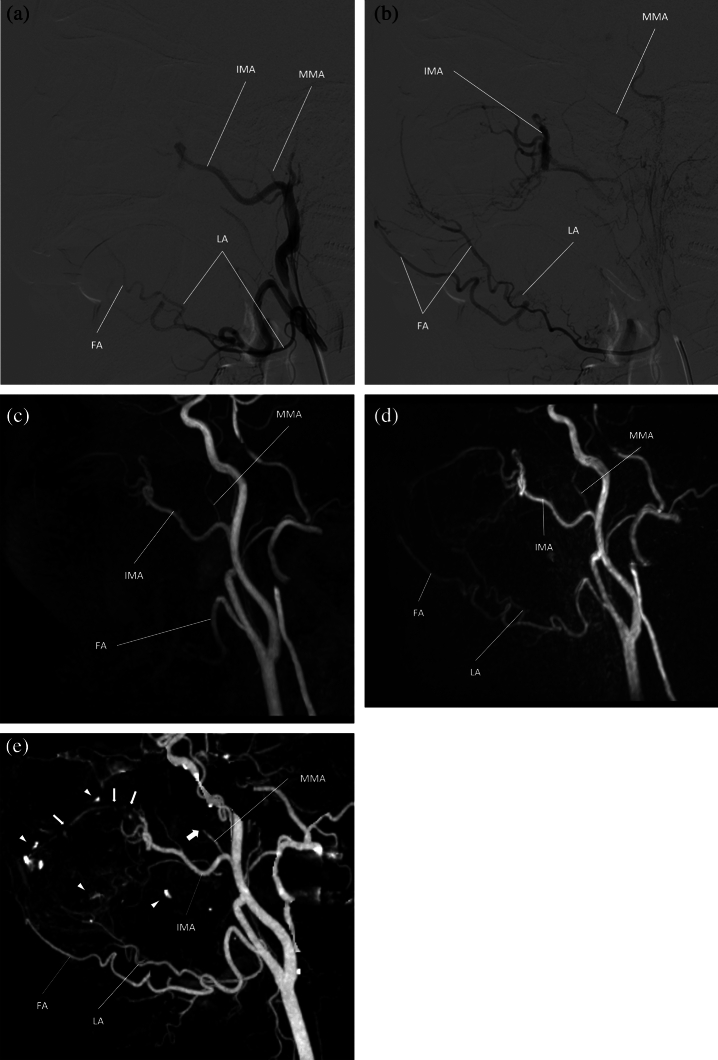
A 67-year-old male with left maxillary sinus cancer. (a, b) Two consecutive phases of the angiogram at the left external carotid artery. (c) On TOF-MRA, the lingual artery (LA) is not visualized (score 1), and the facial artery (FA) is visualized over the branching point of the ascending palatine artery, but the branching point of the submental artery (SMA) is not visualized (score 3). Branches of the 3rd segment of the internal maxillary artery (IMA) is partially demonstrated (score 5). The middle meningeal artery (MMA) is well visualized. (d) On ASL-MRA, the LA is demonstrated almost completely (score 5), and the FA is demonstrated over the branching point of the SMA (score 4). More branches of the 3rd segment are visualized (score 5), and the MMA is well visualized (score 5). (e) CTA demonstrated the LA and FA clearer than both MRAs (score 5 and 4, respectively). Note that the extent of visualized FA is the same as that on ASL-MRA. CTA demonstrates the branches of the 3rd segment as well as ASL-MRA (score 5); however, the visualization of the 3rd segment is inferior to ASL-MRA, because there are some disruptions (thin arrows) and some spots of contrast enhancement (arrow heads). For the MMA, only the origin is visualized on CTA (thick arrow).
